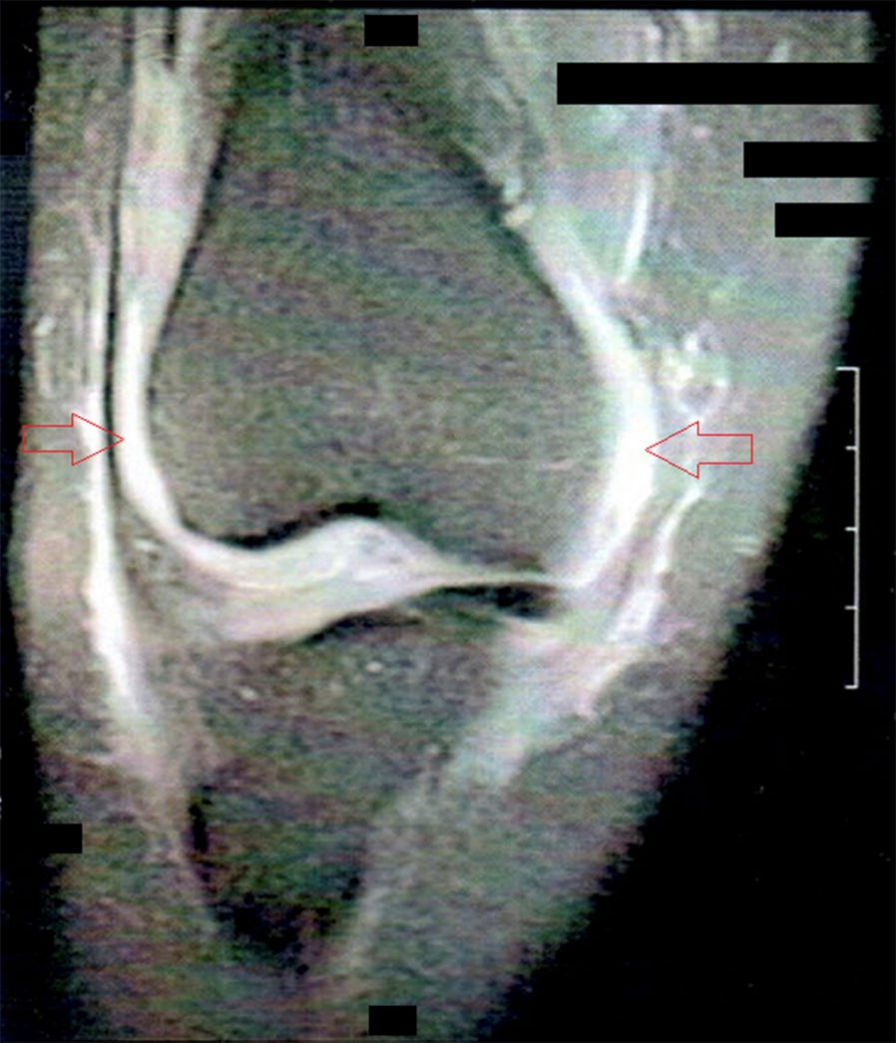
Right knee magnetic resonance image: joint effusion with synovitis signs. Patient presented with intense pain on the right knee, with increased capitation in bone scintigraphy, medial compartment tibiofemoral joint space narrowing and suprapatellar synovial bursa opacification in the radiograph. The magnetic resonance image showed joint effusion and signs of synovitis, indicated by *both arrows in the figure*, without any other alteration, along with the clinical picture, indicate active arthritis
